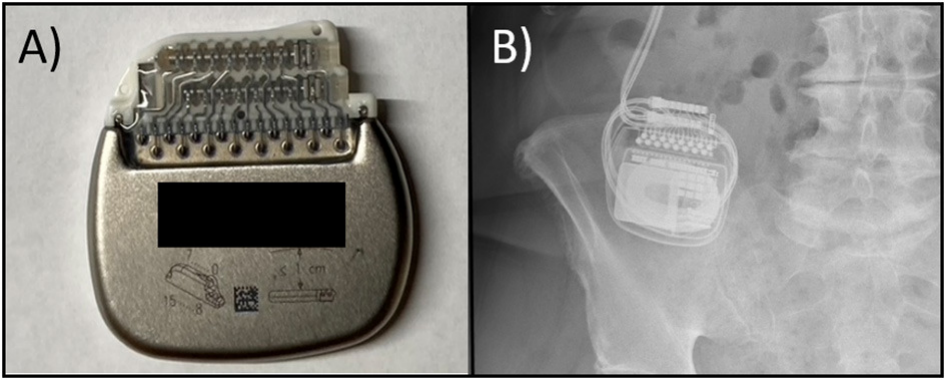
(A) Photo of a rechargeable IPG with the expected normal positioning of the DBS leads which exit to the right of the device; the device label is noted to have an upward orientation with the manufacturing label intentionally obscured, (B) A plain film X-ray series from our case demonstrating the abnormal insertion of the leads on the left side suggestive of flipping.
